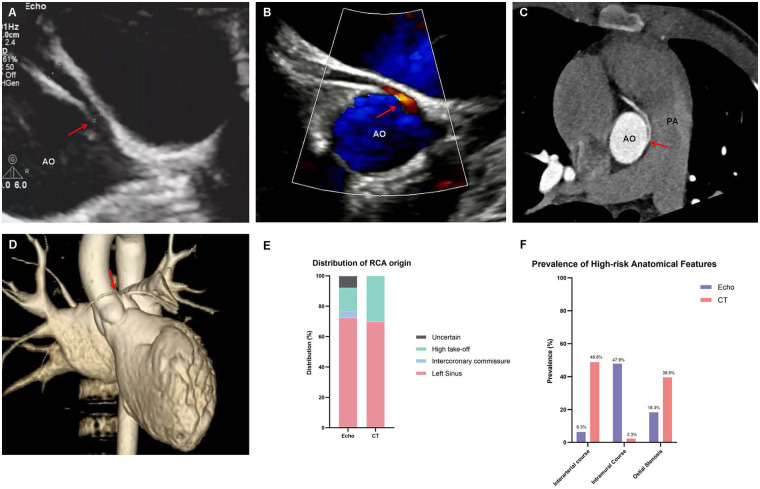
Representative imaging findings and distribution of anatomical features in pediatric patients with AAORCA. (A) Transthoracic echocardiographic image demonstrating an intramural course of the right coronary artery (RCA) with proximal ostial narrowing (red arrow). (B) Color Doppler echocardiography demonstrating accelerated flow at the proximal intramural RCA segment (red arrow), indicating ostial stenosis. (C) CT image showing a high take-off RCA with an interarterial course between the aorta and pulmonary artery (red arrow). (D) CT reconstruction demonstrating anomalous origin of the RCA from the left coronary sinus with ostial stenosis (red arrow). (E) Distribution of RCA origin assessed by echocardiography and CT. (F) Prevalence of imaging-derived anatomical features associated with potential clinical risk, including interarterial course, intramural course, and ostial stenosis, across imaging modalities. LCA, left coronary artery; AO, aorta; PA, pulmonary artery; Echo, echocardiography; CT, computed tomography; RCA, right coronary artery.
